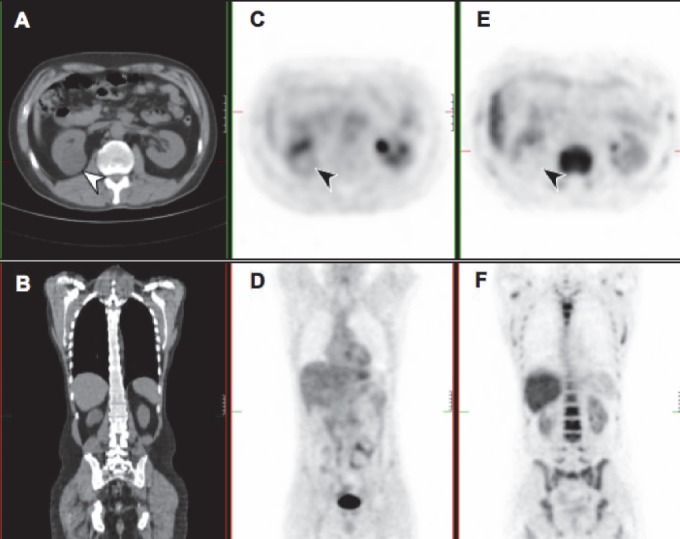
Transaxial and coronal views of a low grade clear cell RCC (arrows). A 35mm mass is seen on the CT image (A, B), with corresponding FDG PET (C, D) and FLT PET (E, F). Uptake in the tumour was less than in surrounding normal kidney
